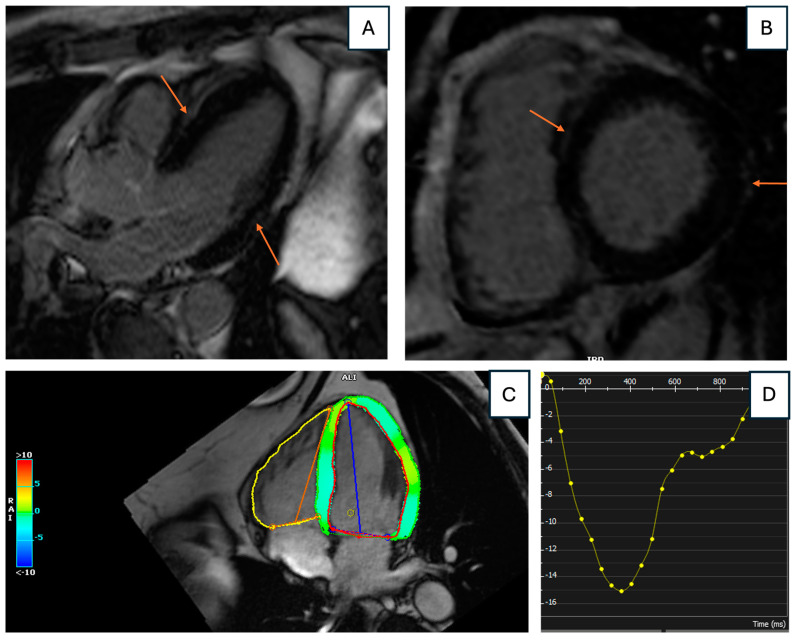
(A) (long-axis view) and (B) (short-axis view) demonstrate cardiac magnetic resonance imaging with subepicardial late gadolinium enhancement (orange arrows) in the basal anteroseptal and inferolateral segments. (C) depicts cardiac magnetic resonance feature tracking global longitudinal strain in four chamber view; (D) depicts left ventricular global longitudinal strain (y-axis) over time during cardiac cycle (x-axis). The peak negative value (–15.0%) indicates a reduced maximal degree of myocardial shortening during systole, suggesting impaired left ventricular systolic function.
